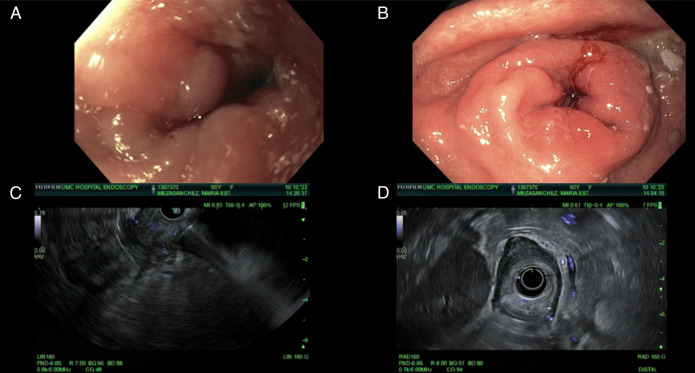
A and B) Endoscopic findings demonstrating severe stenosis in the prepyloric region of the stomach. (C and D) Demonstrate endoscopic ultrasound findings of localized wall thickening in the prepyloric region of the stomach.
